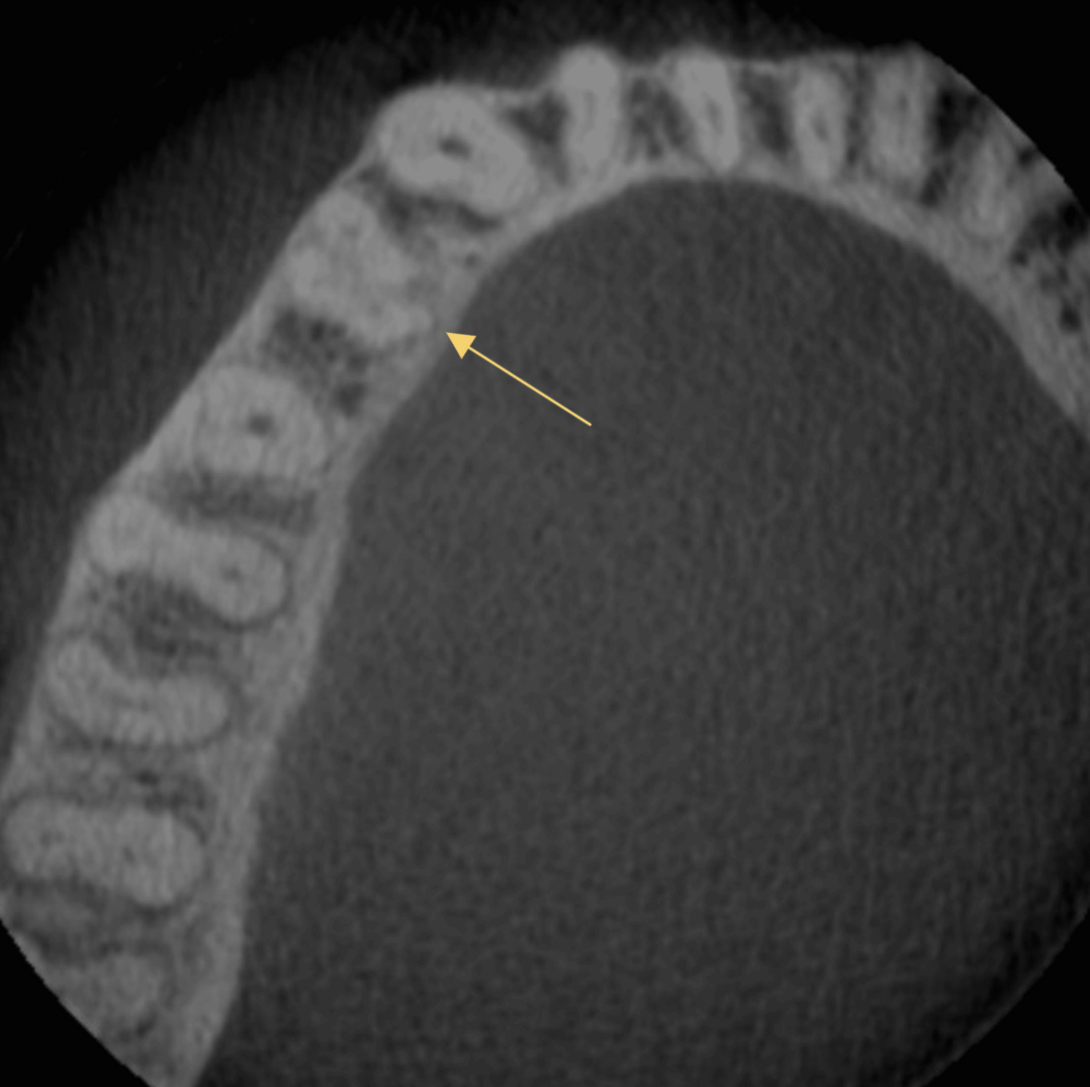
CBCT image of mandibular right first premolar of case 2
